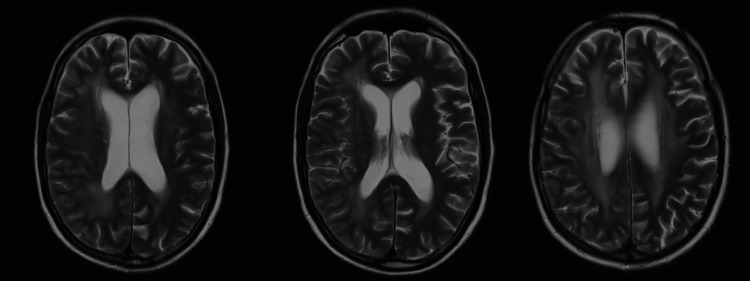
MRI head showing chronic small-vessel ischemia The MRI brain shows global cerebral atrophy, extensive periventricular hyperintensities consistent with chronic small-vessel ischemia, and no evidence of acute infarction or mass lesion
